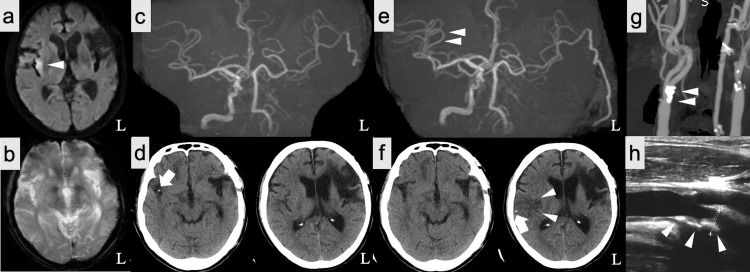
Imaging findings of calcified cerebral emboli in this case a: Diffusion-weighted imaging reveals a high-intensity lesion in the right middle cerebral artery (MCA) territory (arrowhead). b: T2*-weighted gradient echo imaging shows no sign of susceptibility vessel in the right Sylvian fissure. c: Magnetic resonance angiography (MRA) on admission shows no major branch occlusion in the right MCA. d: Axial computed tomography (CT) images reveal a high-density spot suspected to be a calcified embolus in the right Sylvian fissure (arrow). e: Follow-up MRA shows recanalization of the right M2 segment (arrowheads). f: Follow-up CT performed 24 hours after intravenous thrombolysis revealed low-density lesions at the middle MCA territory (arrowheads) and migration of the calcified embolus to the distal MCA (arrow). g: CT angiography shows calcified plaque at the cervical segment of the right internal carotid artery (ICA) (arrowheads). h: Carotid ultrasonography shows calcified plaque at the cervical segment of the right ICA (arrowheads).
